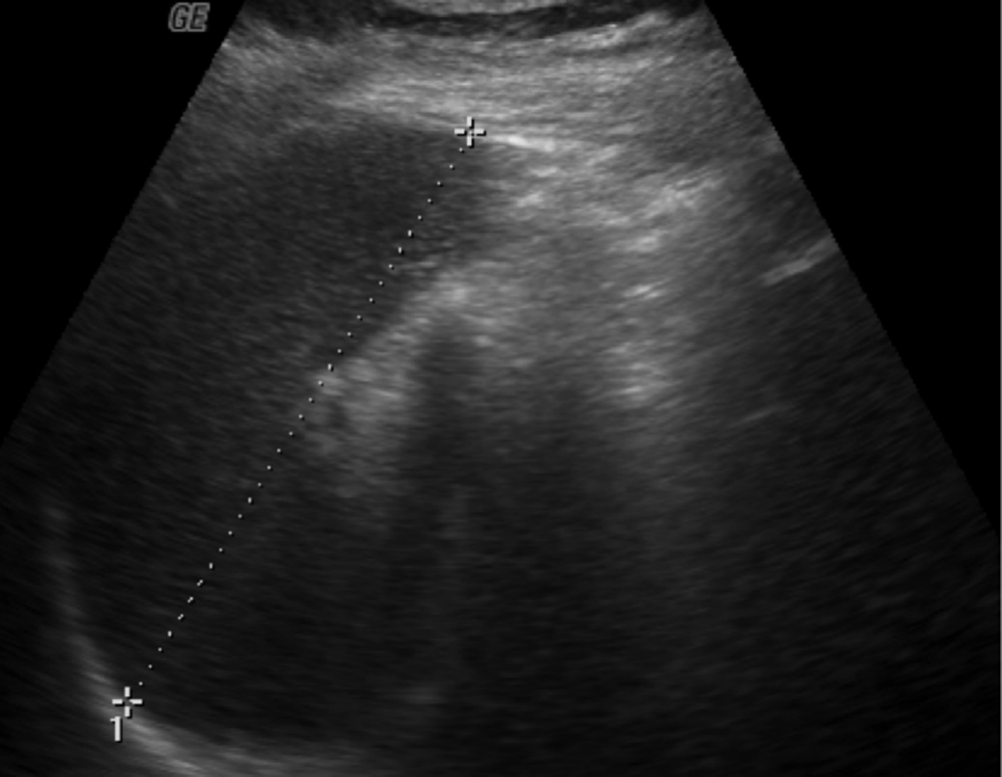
Ultrasound image of spleen showing normal echotexture of spleen without any focal nodular lesions.
